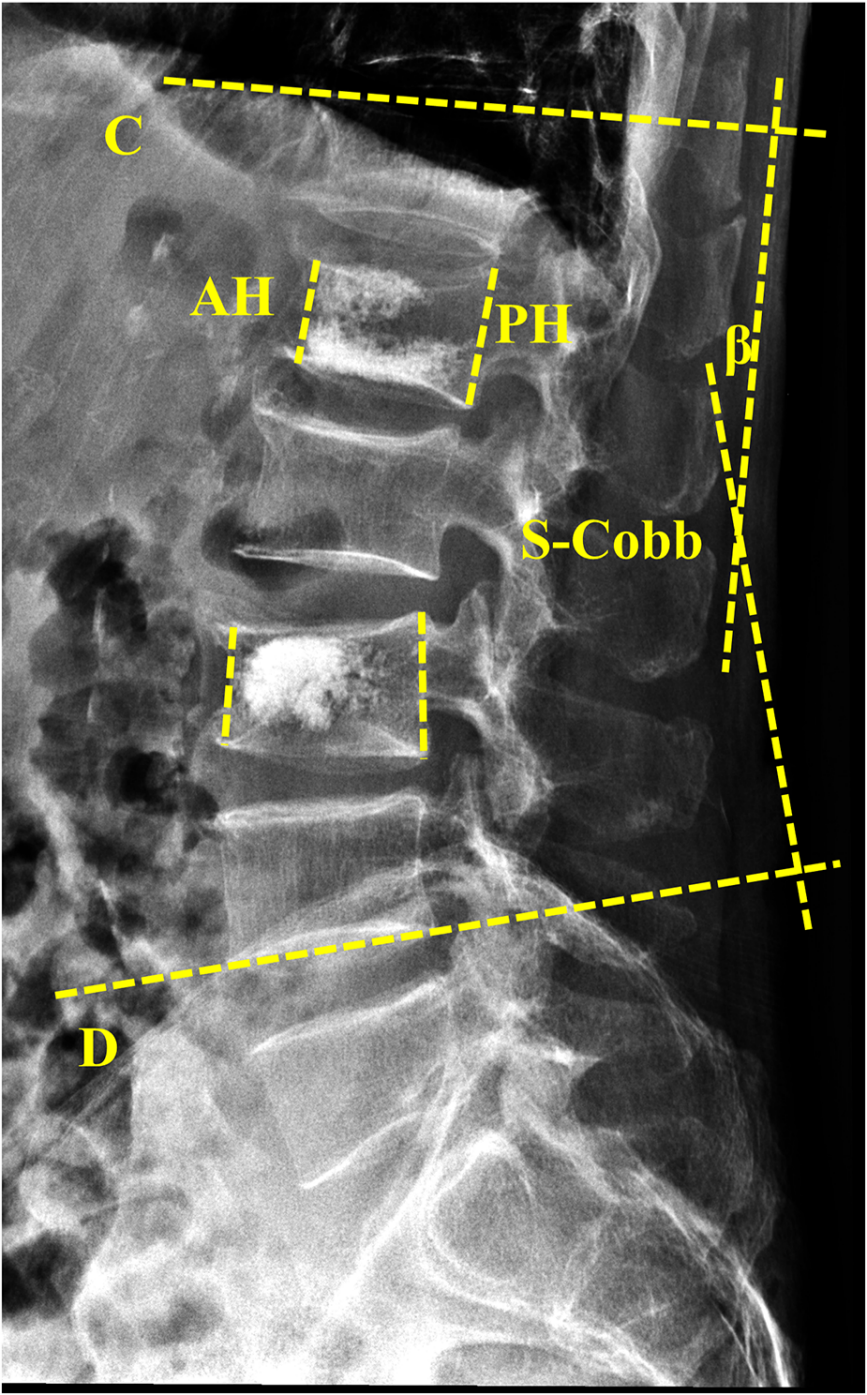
Measurements of sagittal segmental cobb (S-cobb) and the vertebral compression ratio (VCR). The heights of both anterior (AH) and posterior (PH) vertebral body were measured through lateral thoracolumbar radiography. The vertebral compression ratio (VCR) was calculated as the following formula: VCR = |PH–AH|/PH. The Sagittal Segmental Cobb (S-Cobb) angle β was measured from lateral thoracolumbar radiograph by recording the angles between line C and line D, which were measured between the inferior, superior adjacent treated vertebras through lateral radiographs.
